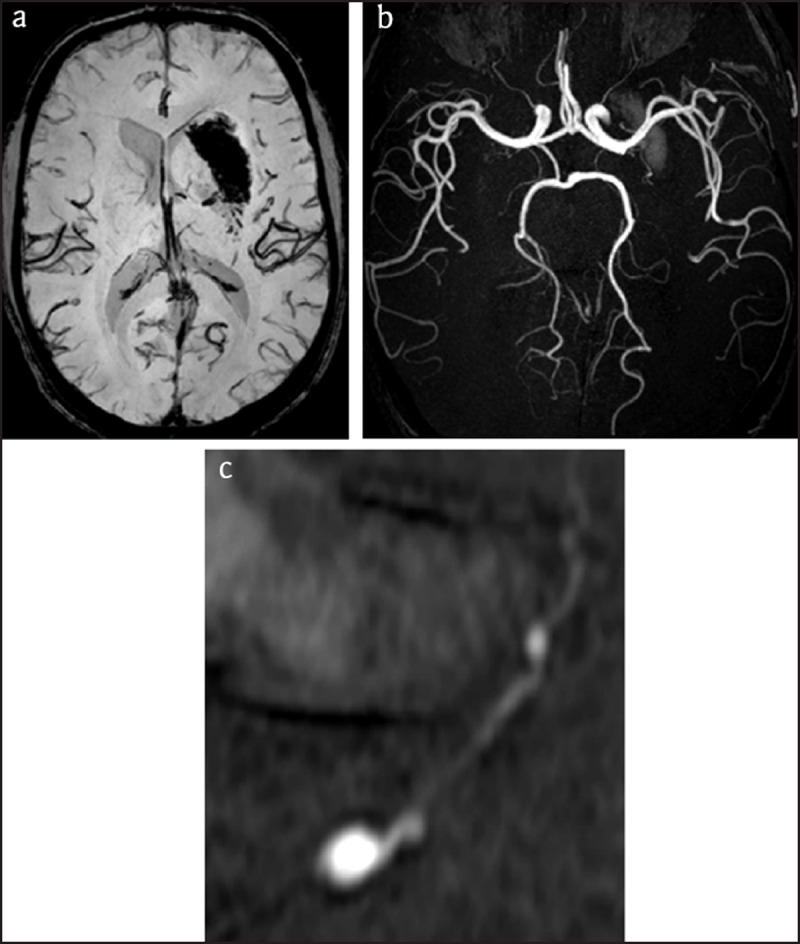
MRI. (a) SWI sequence of MRI: Susceptibility artifacts in the left lentiform nucleus due to hemorrhage. (b) MRA with TOF sequence: Notice aneurysm as a dense spot (arrow) in the T1 hyperintense hemorrhage. (c) MIP of MRA with reconstruction: Notice fusiform dilatation of distal lenticulostriate artery (arrow).
